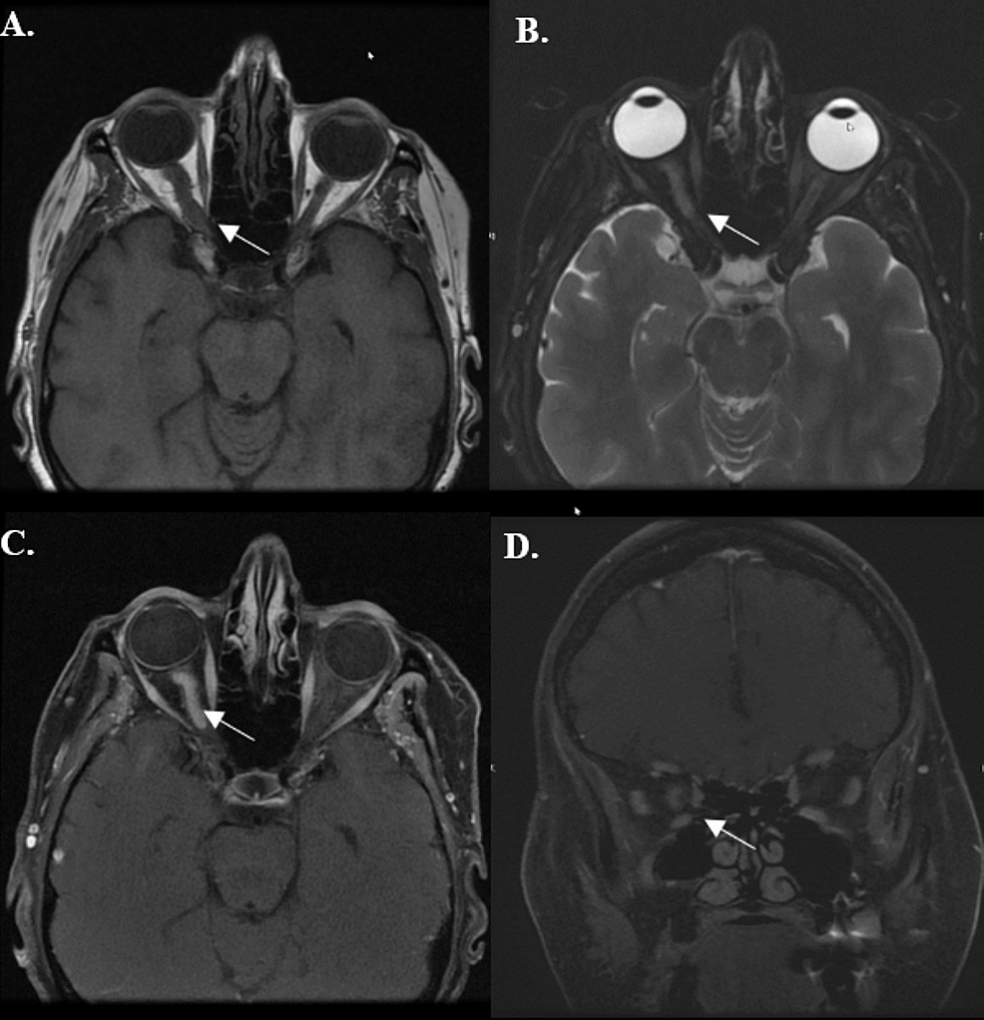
MRI images of the orbits one month prior to the start of second course of radiation treatment (RT2). A) Axial T1 fast spin echo (FSE) sequence. B) Axial T2 FSE sequence, demonstrating diffuse T2 hyperintensity along the intraorbital segment of the right optic nerve. C) Post-contrast axial T1 FSE sequence, demonstrating enhancement along the intraorbital portion of the right optic nerve. D) Post-contrast coronal FSE sequence, demonstrating near circumferential involvement of the right optic nerve by optic nerve sheath meningiomas (ONSM).
